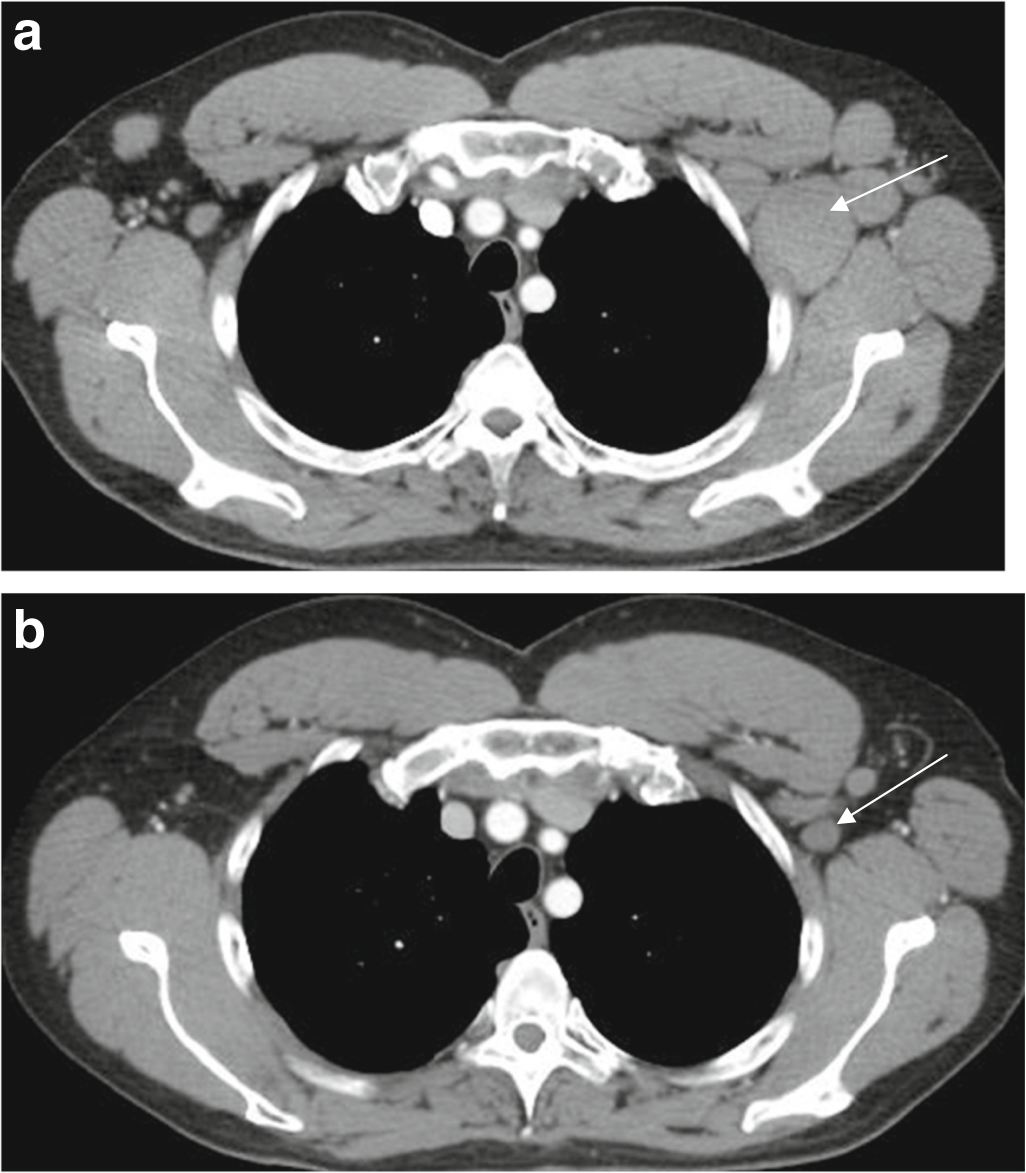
59-year-old male with follicular lymphoma was observed initially for 6 months after diagnosis but he progressed with increasing left axillary lymphadenopathy. a Axial CT images of the chest acquired during arterial phase reveal left axillary lymphadenopathy with the largest lymph node measuring 5 cm in short axis (white arrow). b Axial CT images of the chest acquired during arterial phase after 1 year on Ofatumumab demonstrate decrease in the size of left axillary lymph node which now measures less than 1 cm in short axis
